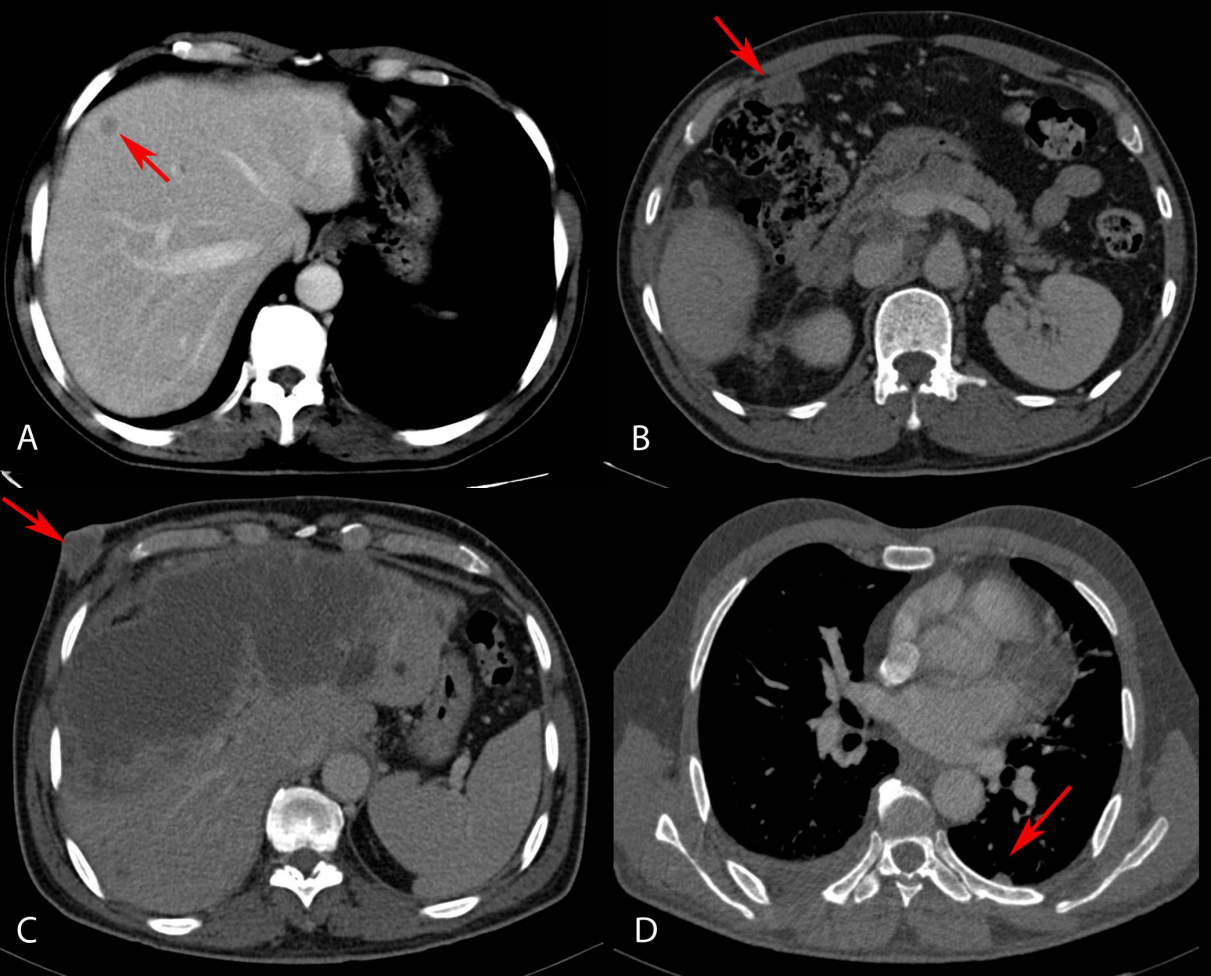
CT images. A) Hepatic metastasis (red arrow). B) Peritoneal metastases (red arrow). C) Liver infiltration, hilum adenopathies, and subcutaneous nodule at the entrance of the right laparoscopy trocar (red arrow). D) Nodular metastases in left pleura (red arrow) and right pleural effusion.
